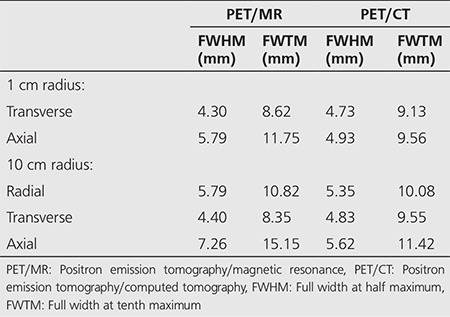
Spatial resolution values in positron emission tomography/magnetic resonance and positron emission tomography/computed tomography
